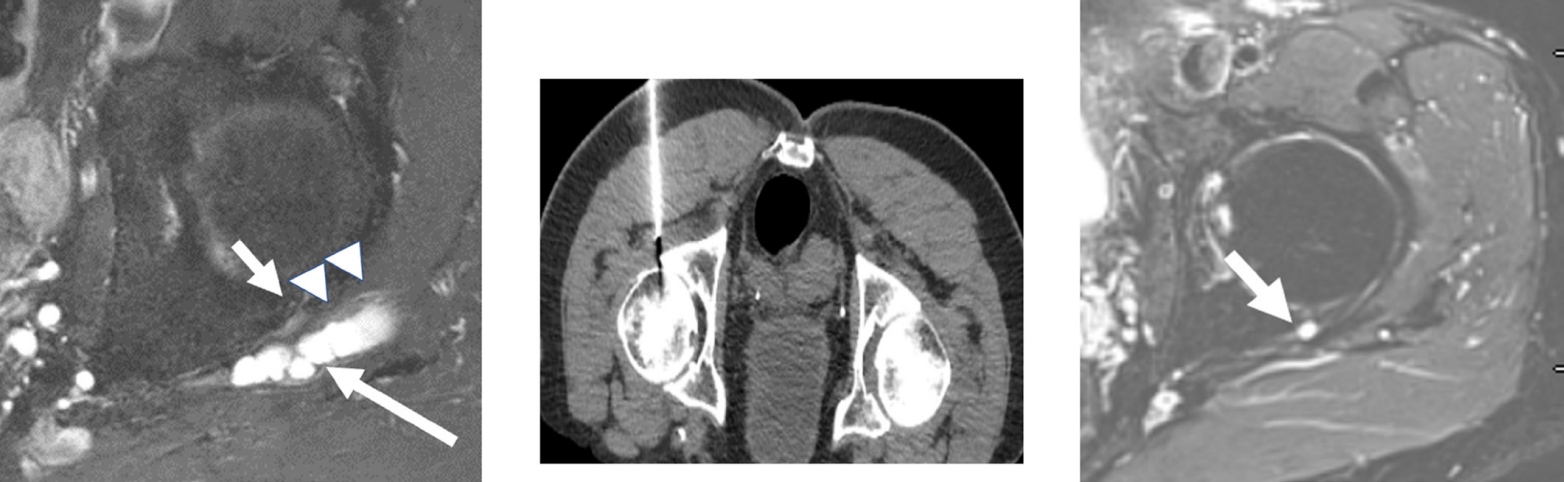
46-year-old female. Figure 2A: Axial fsPDW image demonstrating a paralabral cyst (long arrow) arising from a posterior labral tear (small arrows) with posterolateral extension adjacent to the sciatic nerve (arrowheads).Figure 2B: CT image in prone position during cyst fenestration and attempted aspiration with 16G needle. Figure 2C. Follow-up axial fsPDW MR of left hip 2 years later shows resolution of the cyst with residual labral tear still present (small arrow). fsPDW, fat saturated proton density-weighted.
